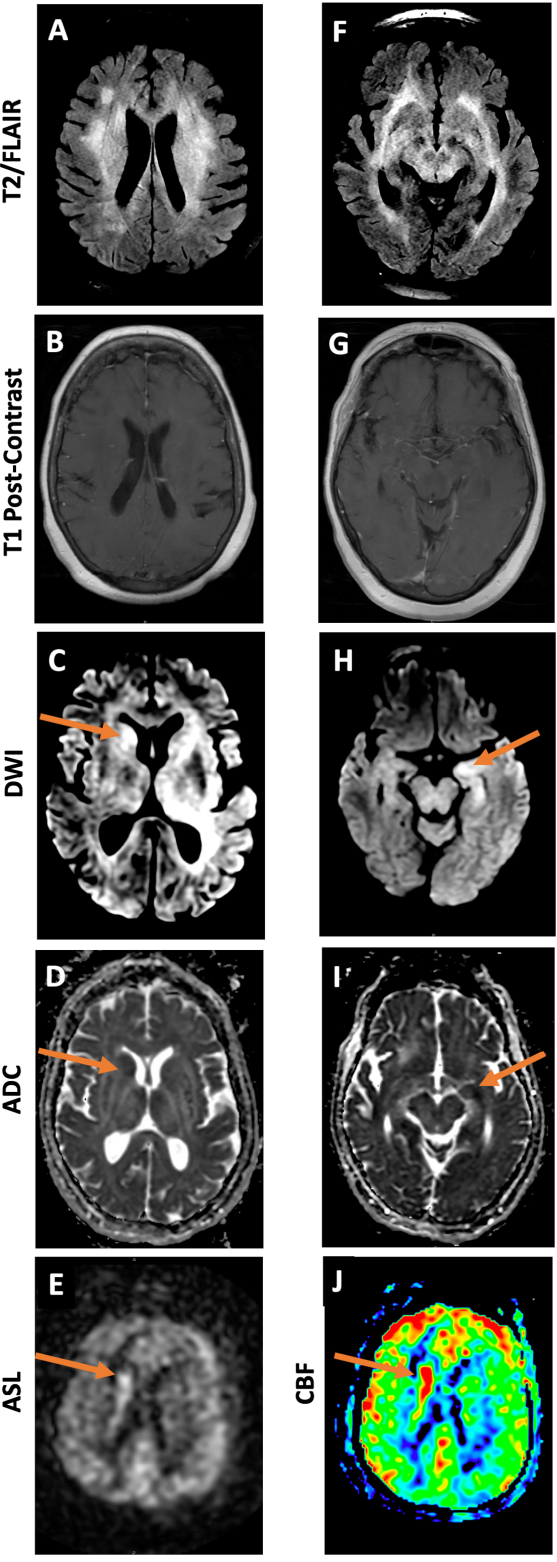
MRI brain 3 months after initial presentation demonstrates diffuse T2/FLAIR hyperintense lesions (A, F). DWI and ADC images demonstrate restricted diffusion in the region of the right caudate (C, D) and left hippocampus (H, I). Grayscale and color ASL perfusion images also demonstrate elevated perfusion in the region of the right caudate (E, J). No abnormal enhancement or mass is seen in either area (B, G).
